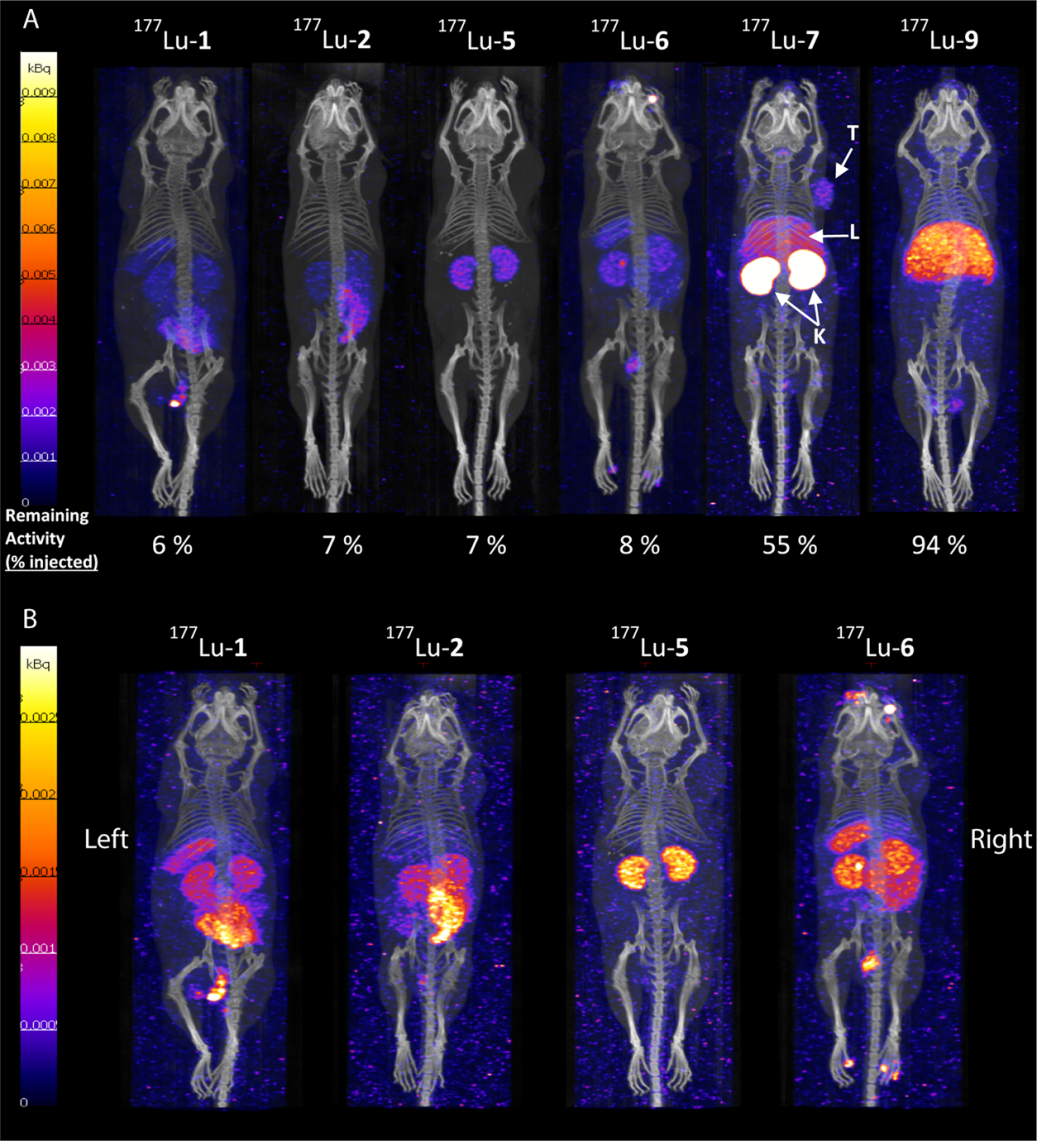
SPECT/CT images as maximum intensity projections
